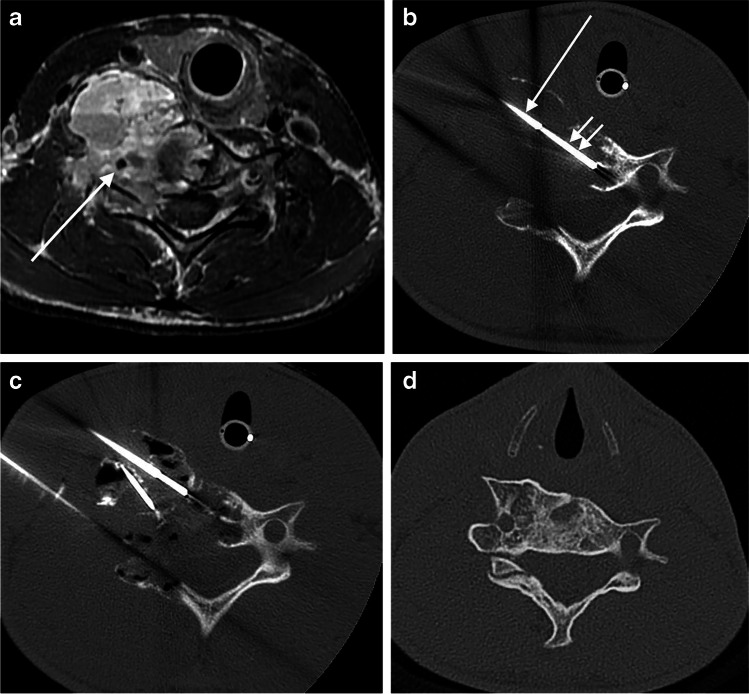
Cross-sectional images from a 14-year-old boy (patient 9) with a C6 aneurysmal bone cyst (ABC). a Axial pre-treatment supine T1-W MR image following contrast administration shows a destructive and exophytic multilocular cystic mass replacing the right half of the C6 ring and surrounding the vertebral artery (*arrow*). b Axial supine CT image during biopsy and first treatment at the same level as (a) shows a 14-gauge (G) guiding needle (*single arrow*) through which passes a 15-G biopsy needle (*double arrows*). c Axial supine CT on the same date and at the same level as in (a and b) shows three separate needles within different portions of the ABC with doxycycline foam (appearing black from air in foam) throughout the different loculations of the lesion. d Diagnostic axial CT at same level 3 years after last treatment shows healing of the ABC
